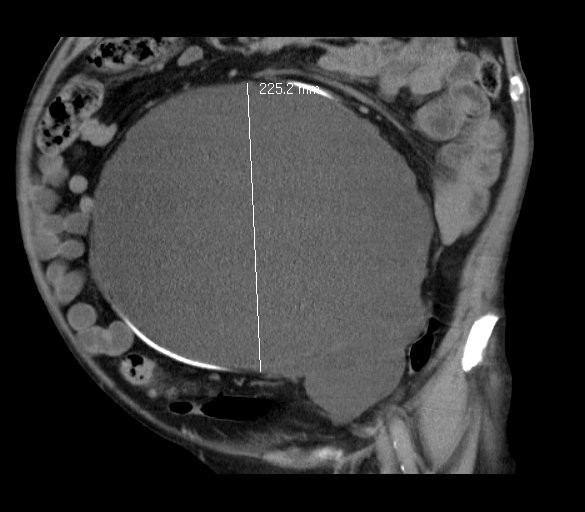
Computed tomography scan of giant lymph cyst.
